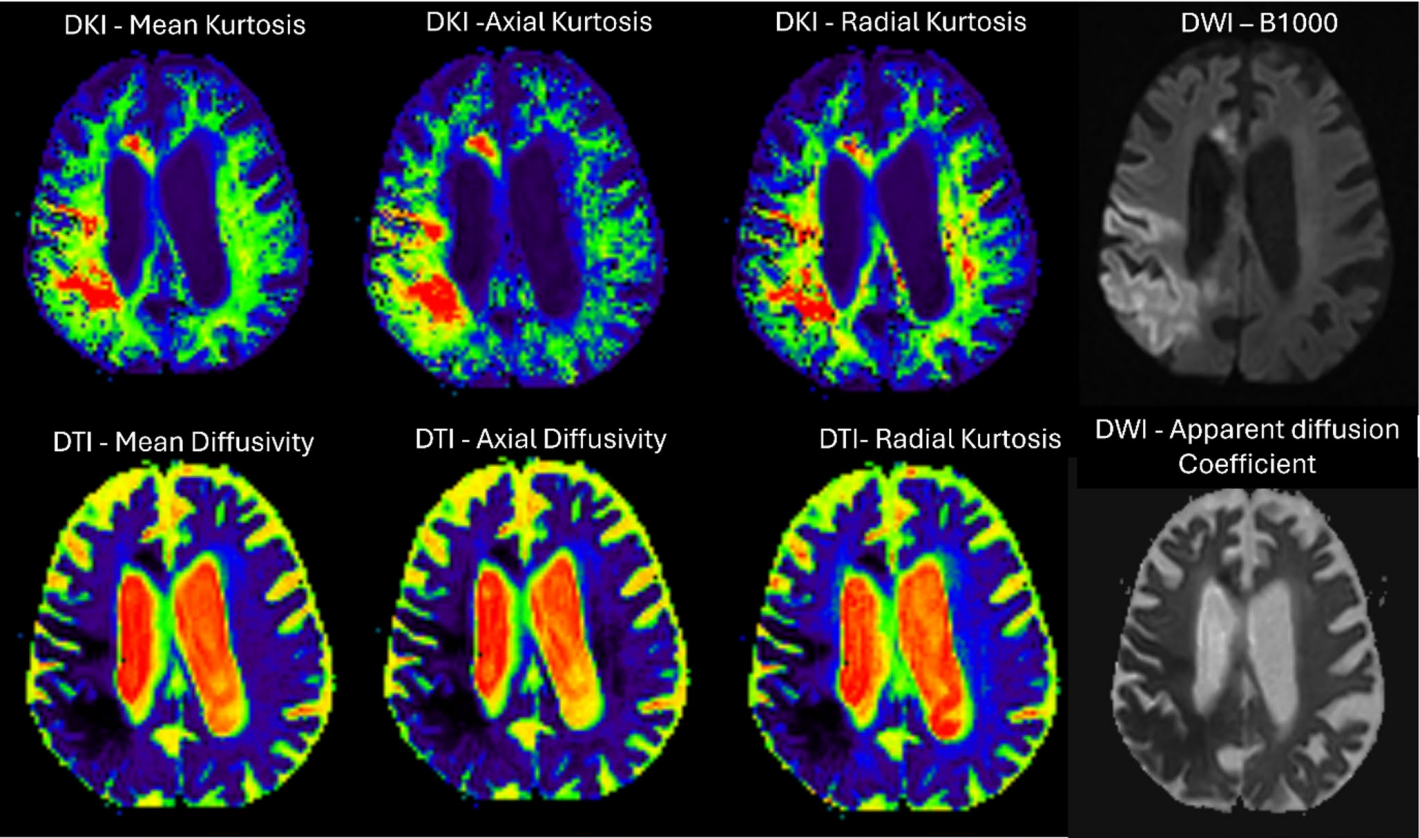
Representative DWI, DTI, and DKI maps of an 86 y/o female with an acute ischemic stroke in the left parietal lobe. Image courtesy of Department of Radiodiagnosis and Imaging, Kasturba Medical College, Manipal. Patient scanned using United Imaging uMR780 3 T scanner
